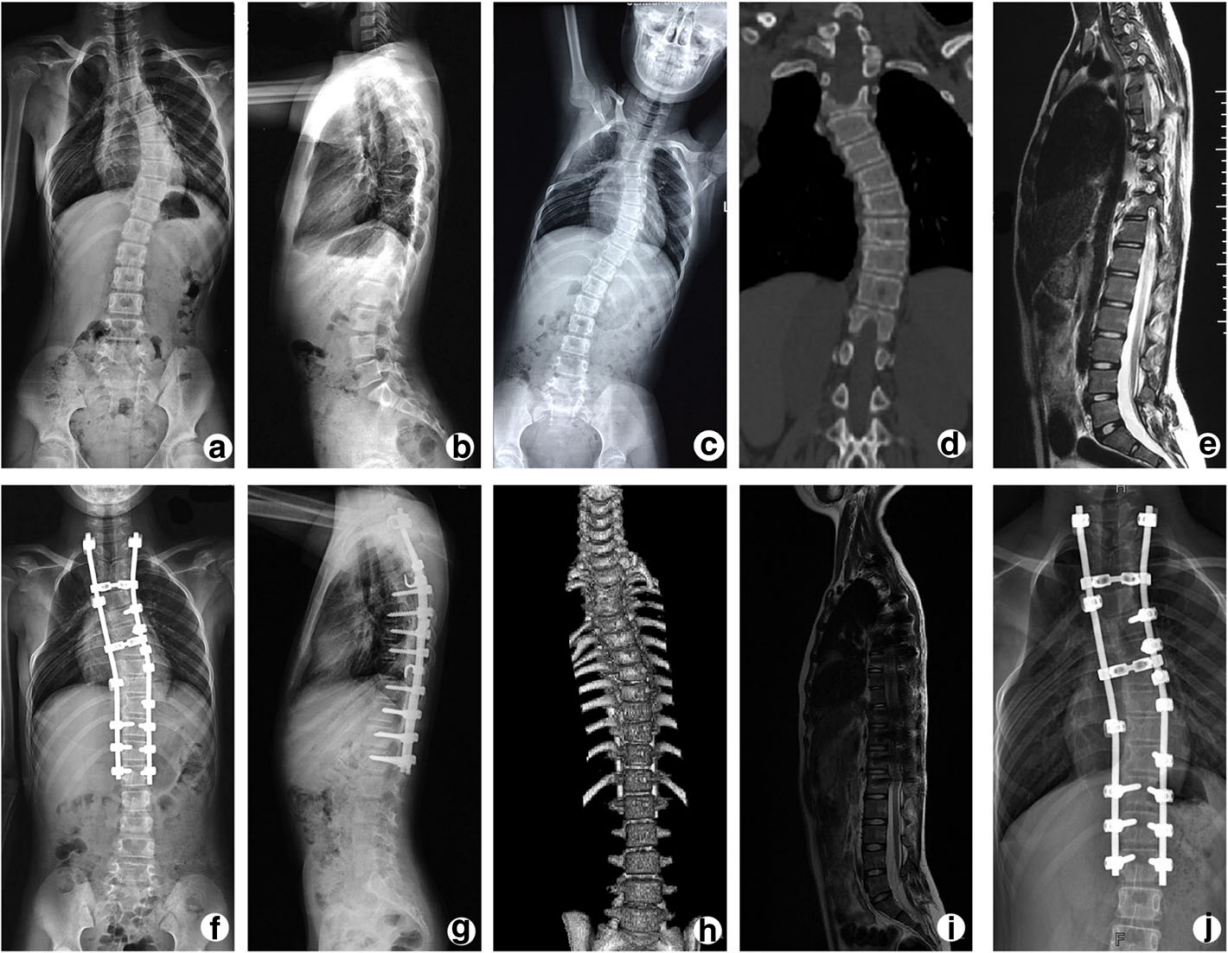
The pre-operative radiograph and CT (Fig. 3
